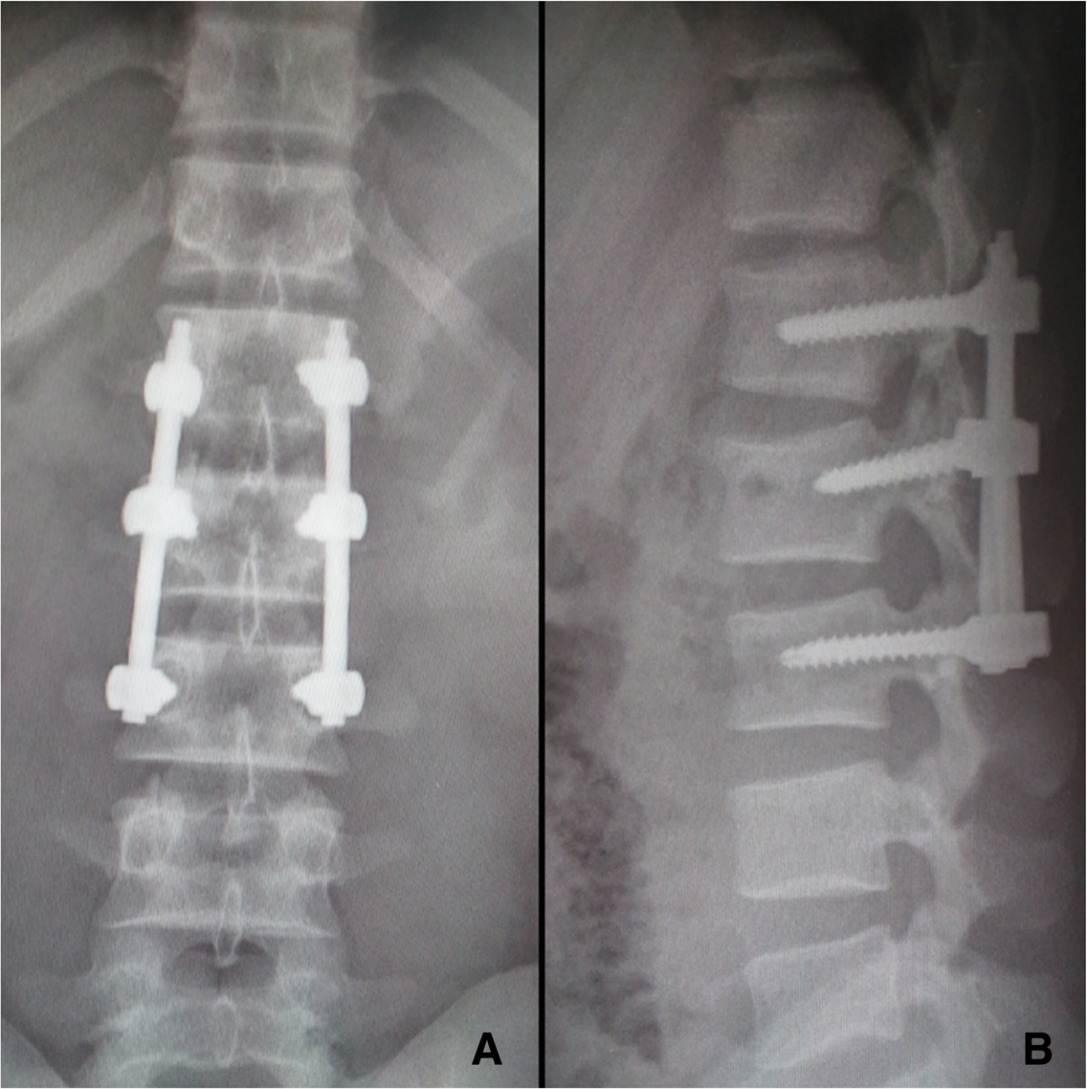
a Anteroposterior and b lateral radiograph of the lumbar spine showed the pedicel screws were inserted in the L1-3 vertebrae
